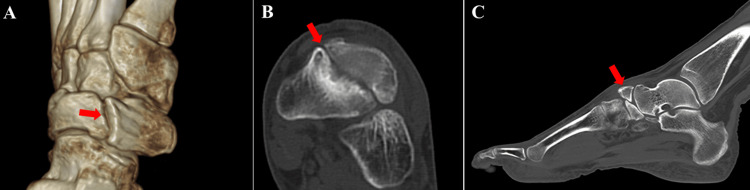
Preoperative computed tomography findings. The bipartite navicular was confirmed (red arrows). (A) 3D image, (B) axial view, and (C) sagittal view.
